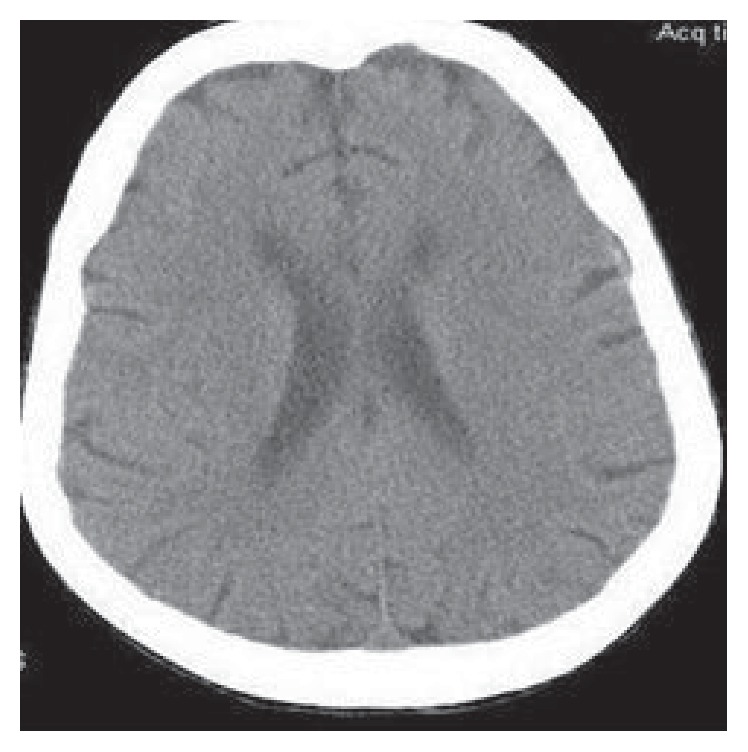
CT scan of head without any intracranial lesion.
